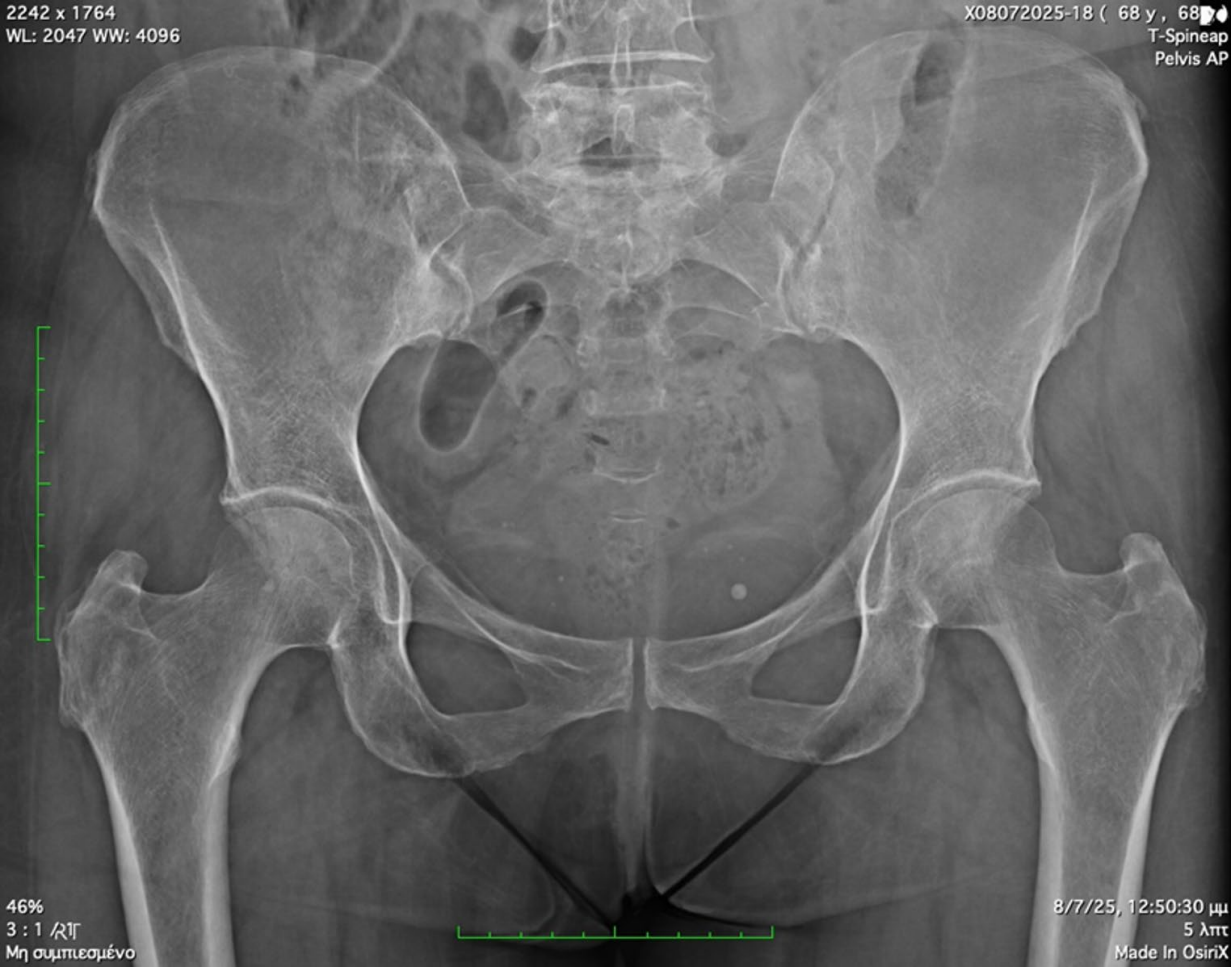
Pelvis x-ray, anteroposterior view. Note that the sacroiliac joints are of uniform width and the cortical lines along joints are intact. The hip joints are also normal.
